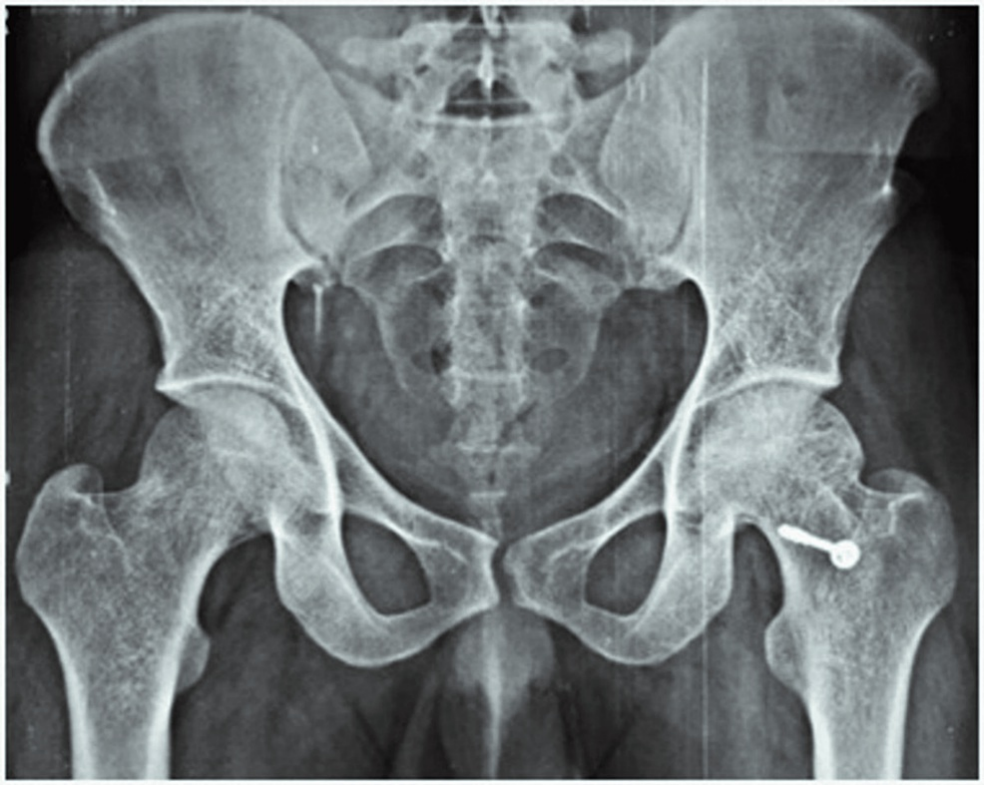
Postoperative radiograph at five years of follow-up
